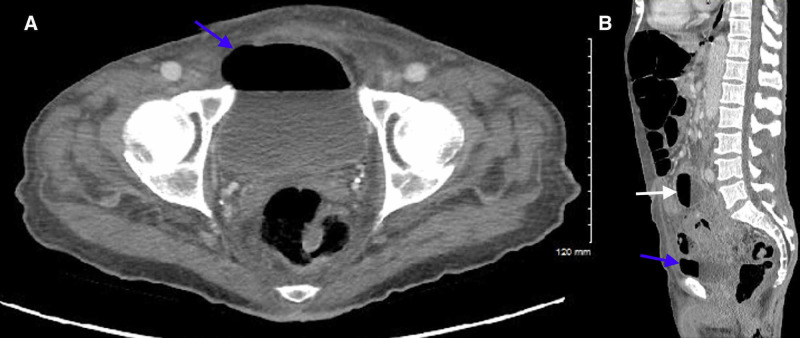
CT of the abdomen/pelvis demonstrating air in the urinary bladder (blue arrows) and intra-abdominal abscess collection #1 (white arrow). Axial (A) and sagittal (B) planes.
